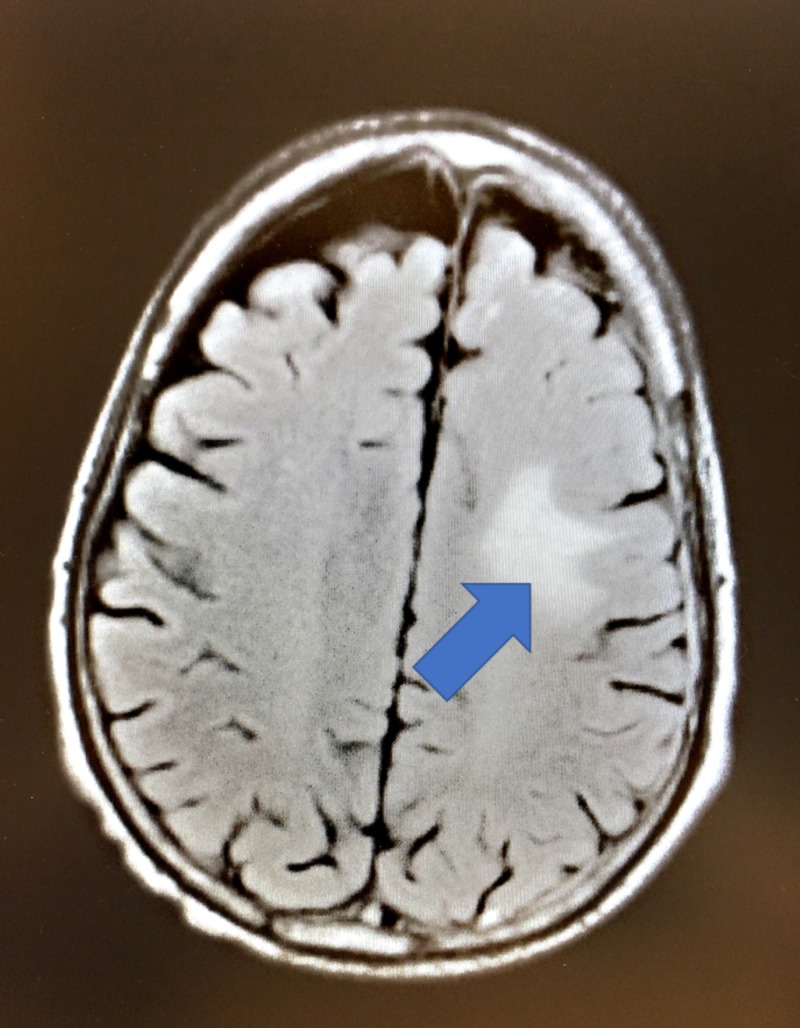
Post-craniotomy MRI showing a stable, small hemorrhage in the left frontoparietal region with persistent associated vasogenic edema. MRI: magnetic resonance imaging
